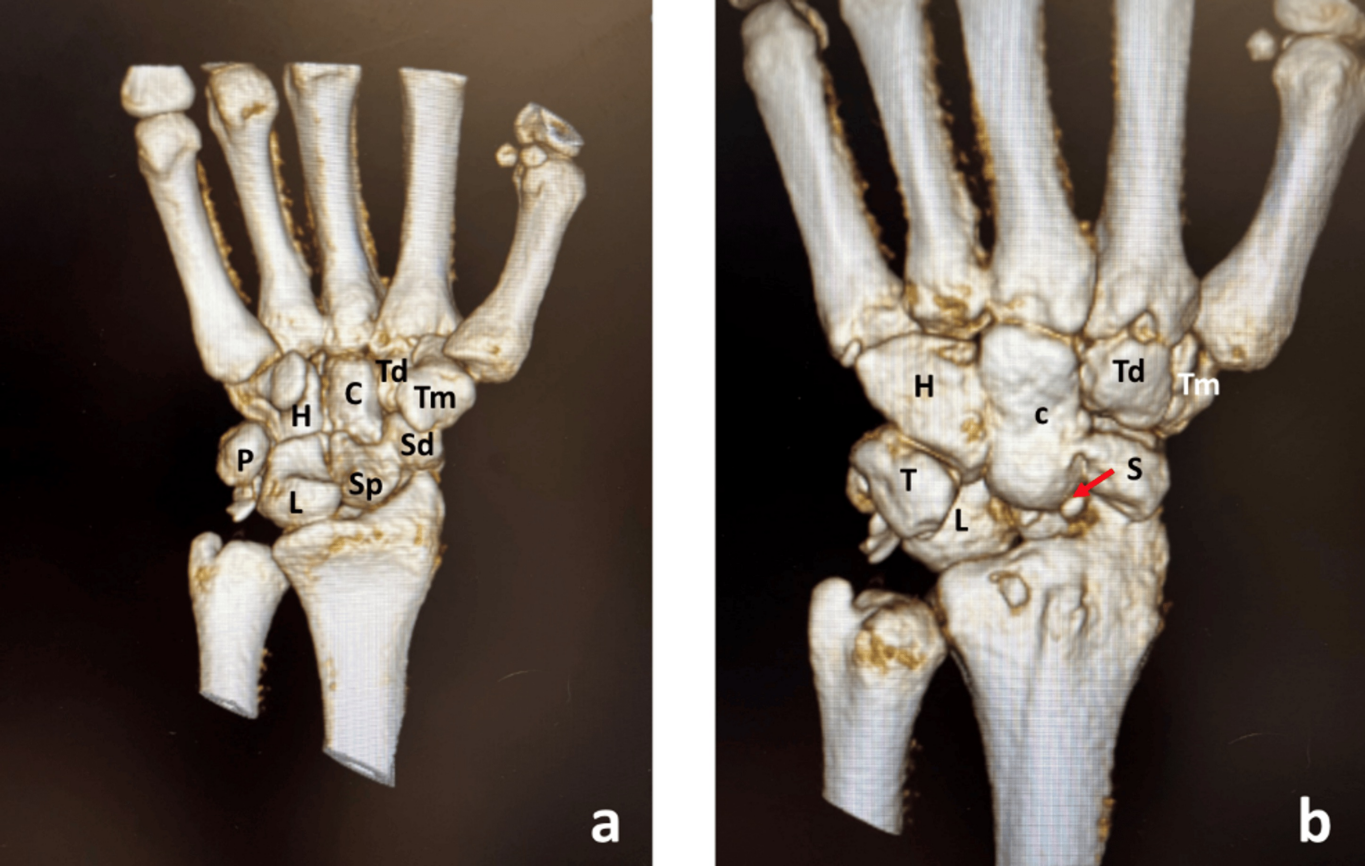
3D CT posteroanterior (a) and anteroposterior view (b). Arrow indicates fractured proximal pole of scaphoid. Sd, distal pole of fractured scaphoid; Sp, proximal pole of fractured scaphoid; L, lunate; T, triquetrum; P, pisiform; H, hamate; C, capitate; Td, trapezoid; Tm, trapezium.
